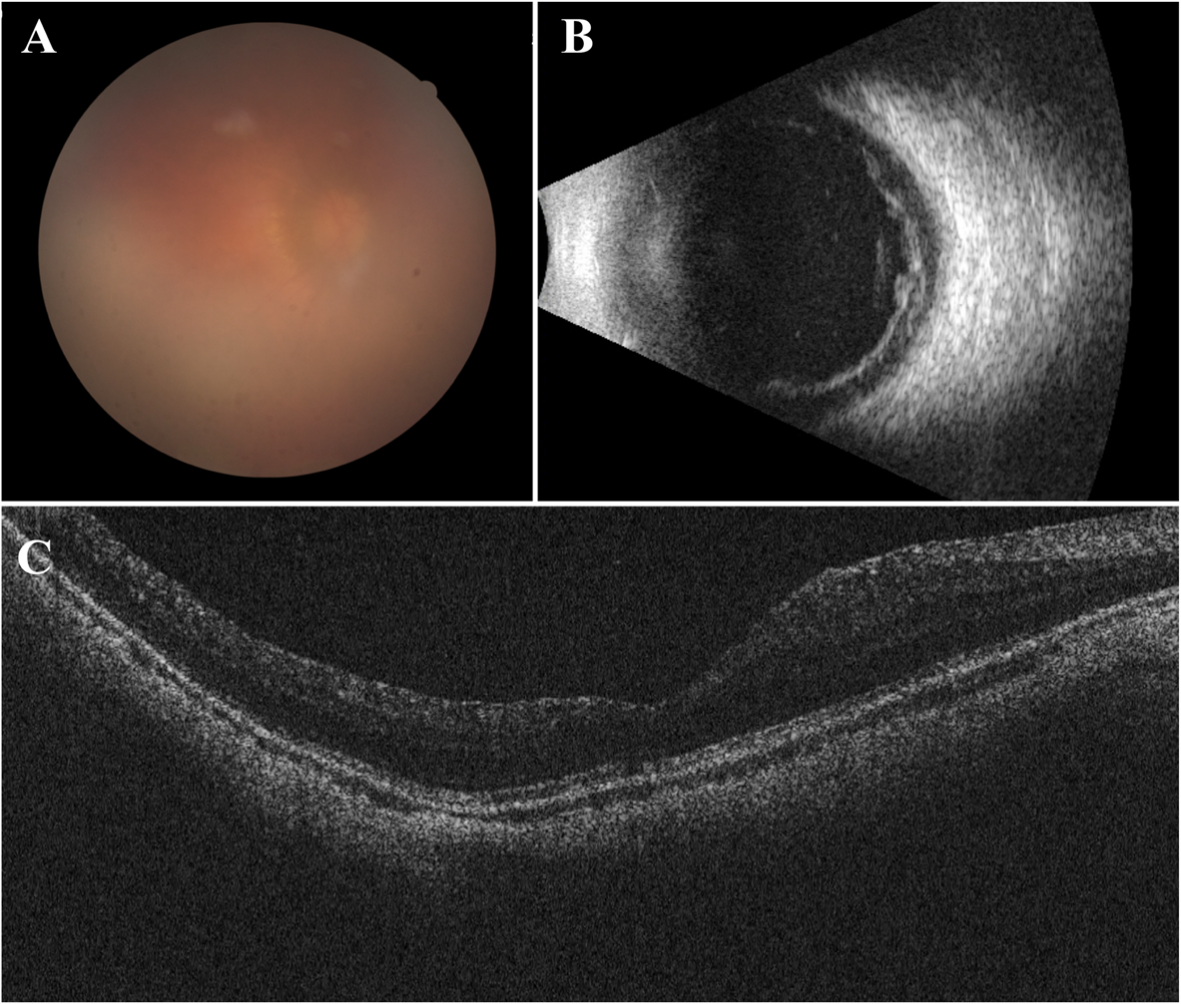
Ophthalmic examinations of the patient at presentation. a Fundus photography showed an opaque image of the posterior segment. b B-scan ultrasound showed a mass of echoes in the whole vitreous cavity and strip-like echoes in the peripheral region. c Optical coherence tomography showed a retinal structure that is common in highly myopic eyes
